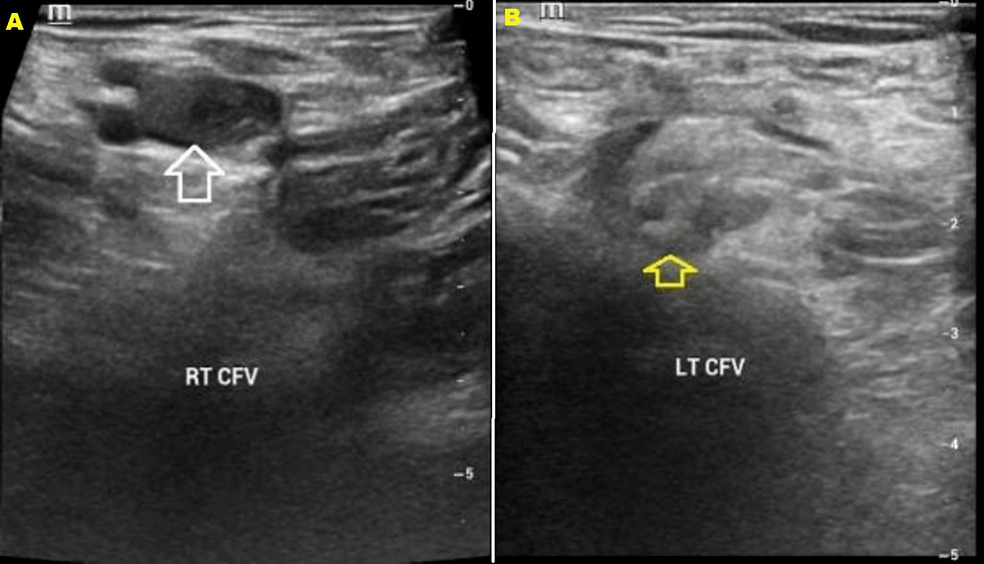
Selected ultrasound images at the level of the right common femoral (A) and left common femoral (B) veins which show a non-compressible vein with an internal filling defect (white and yellow arrows).
